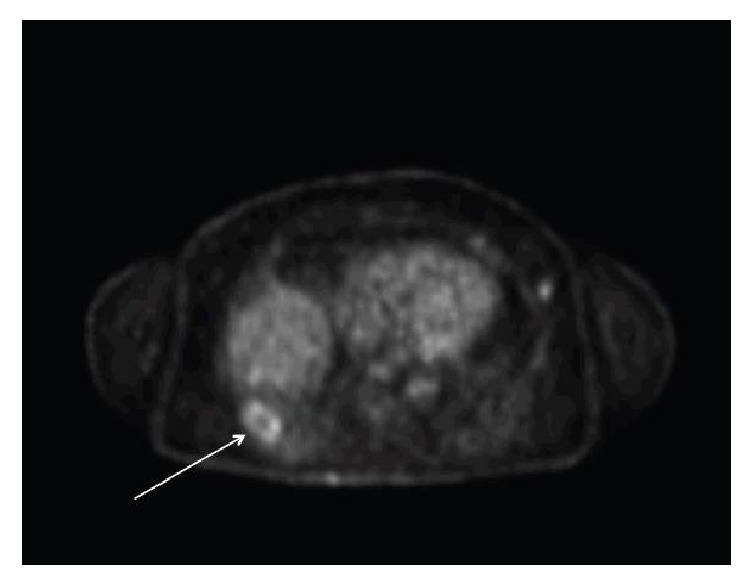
PET scan identifies costophrenic lung mass as peripherally hypermetabolic with relatively ametabolic central region, likely cavitation from necrosis (max SUV 3.9).
